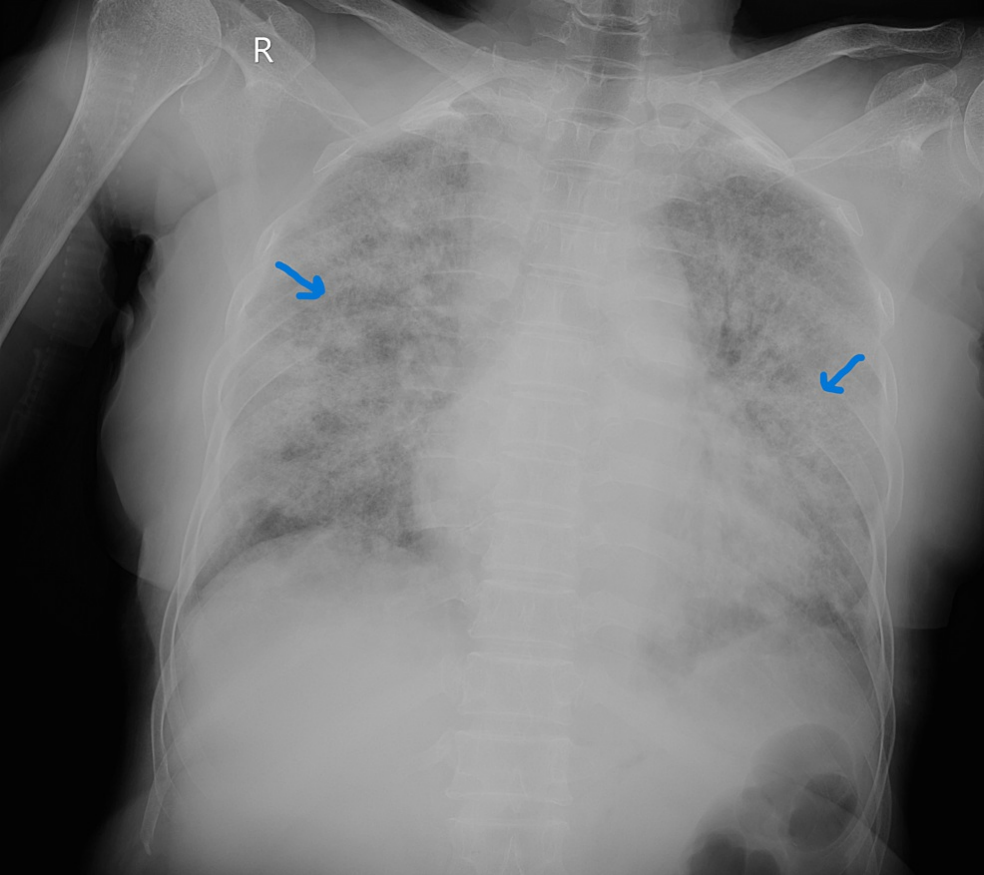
Chest x-ray image showing bilateral patchy lung consolidations
